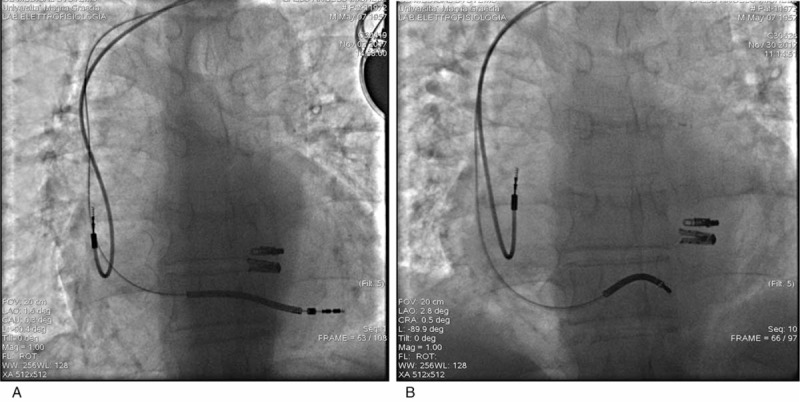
A, Anteroposterior radiographic view showing defibrillator lead in the left ventricle. B, Antero-posterior radiographic view showing defibrillator lead replaced in the apex of the right ventricle after extraction procedure.
